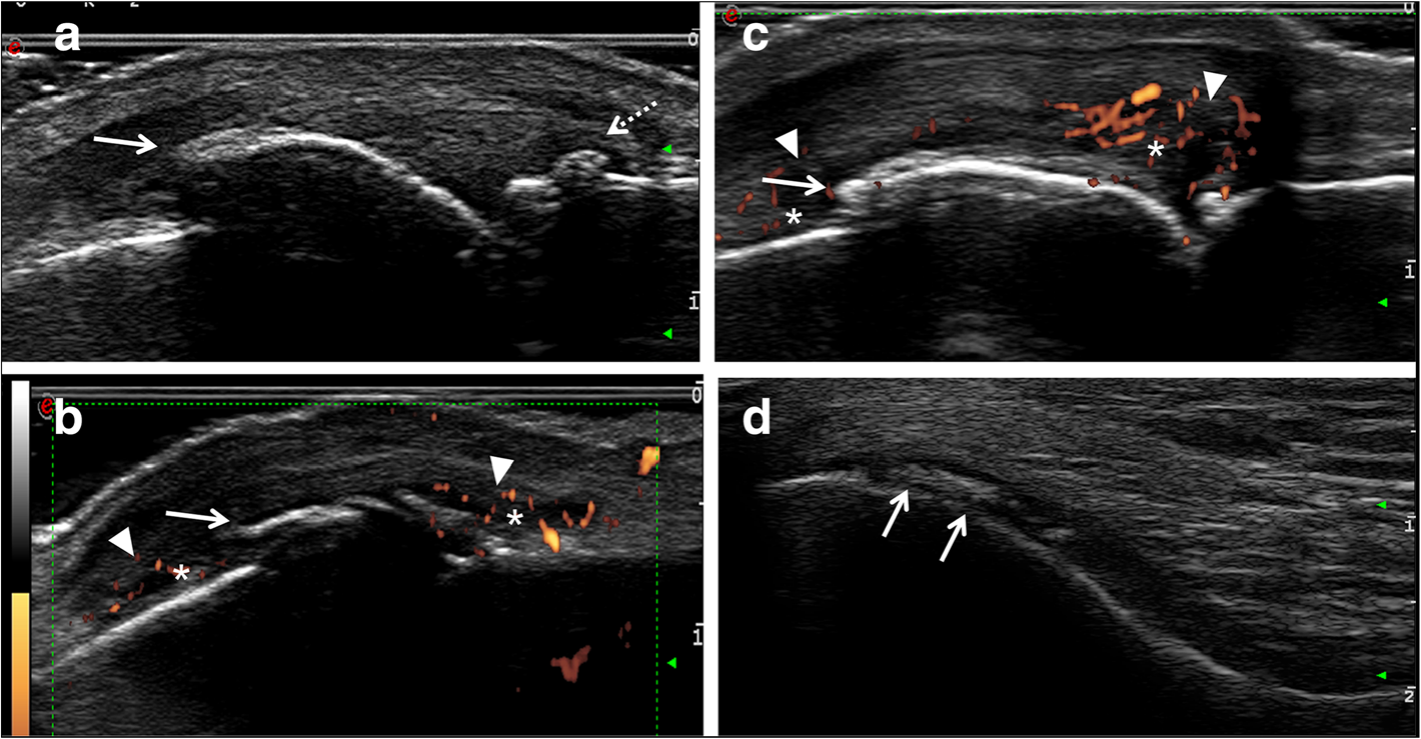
Ultrasound image examples of patients with hereditary haemochromatosis. a Longitudinal dorsal scan of a metacarpophalangeal (MCP) joint (left = proximal) indicating an extensive proximal osteophyte (arrow) and a smaller osteophyte in the distal part of the joint (broken arrow). b, c Longitudinal dorsal scan of MCP joints indicating a proximal osteophyte (arrow), grey scale synovitis (arrow heads) and power Doppler signals (asterix). d Transverse suprapatellar scan of a knee indicating calcium pyrophosphate deposition in the hyaline cartilage (arrow)
